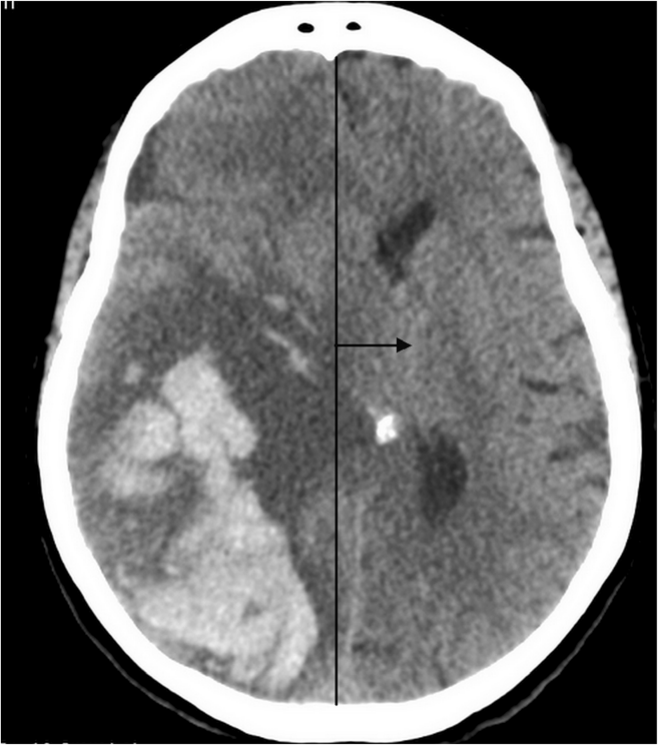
Cranial CT scan. Right parietal occipital hematoma with cerebral oedema and left displacement of the medial brain structures (Black arrow).
